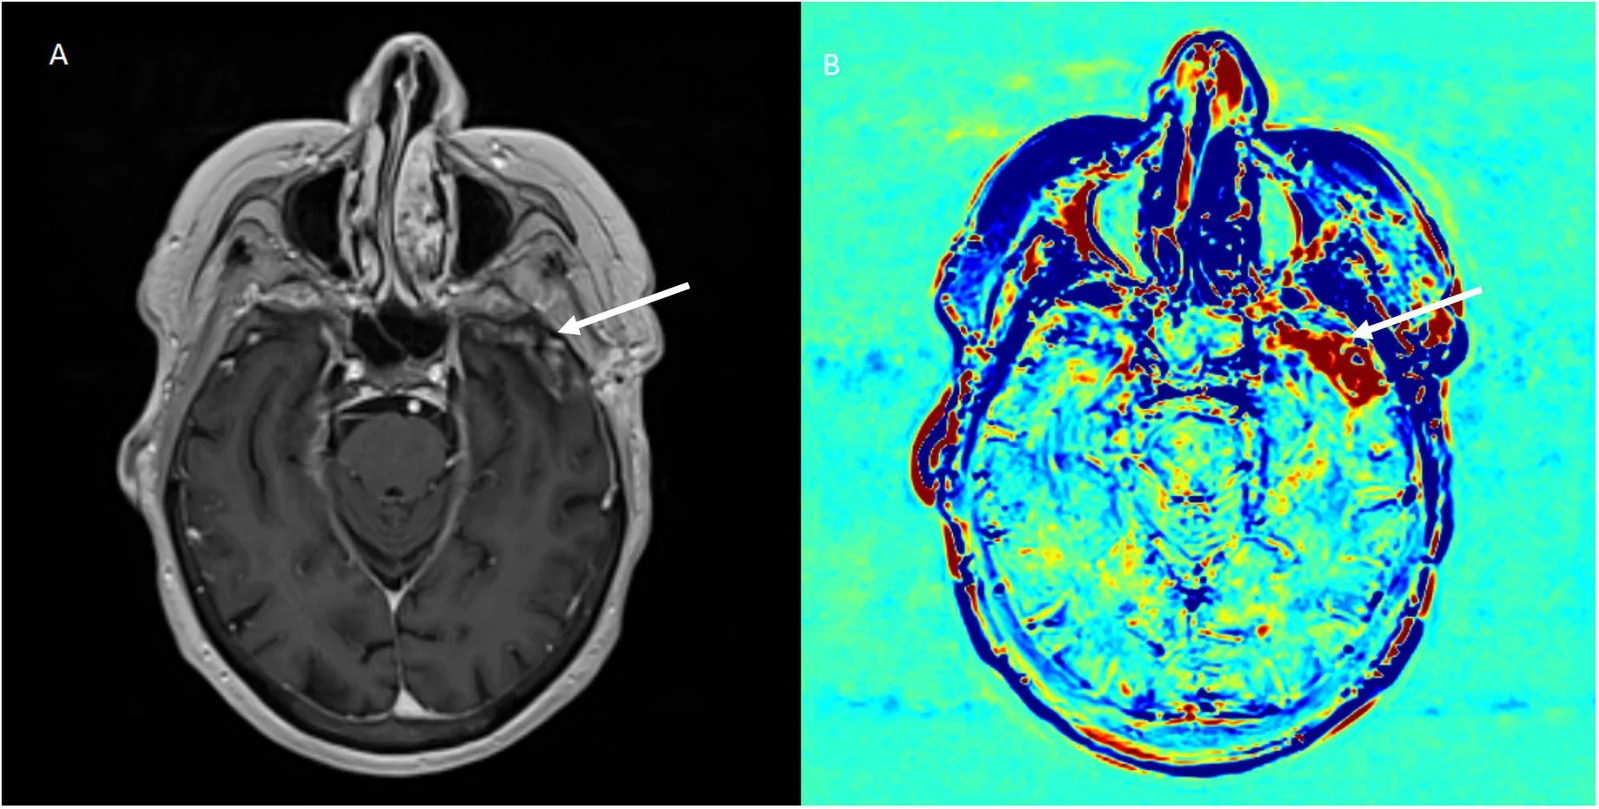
Post-operative TRAMs/CCA findings demonstrating persistent delayed contrast retention without early contrast clearance within the contrast enhancement on the edge of the resection cavity. (A) Contrast-enhanced T1-weighted MRI acquired for SRS planning 2 months after resection demonstrating extensive post-operative contrast enhancement. (B) TRAMs/CCA demonstrating persistent delay contrast retention without early contrast clearance.
